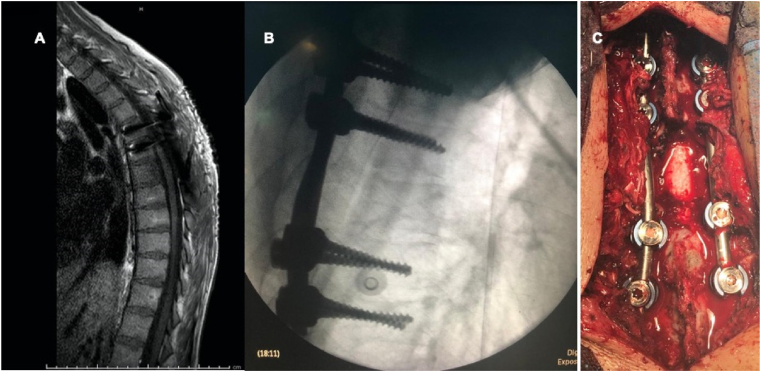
(A) sagittal MRI demonstrates segmental spinal instrumentation with T5-T9 transpedicular screws fusion. (B) lateral plain XRAY demonstrate T5-T9 transpedicular screws fusion. (C) intra-operative view of T5-T9 transpedicular screws fusion.
